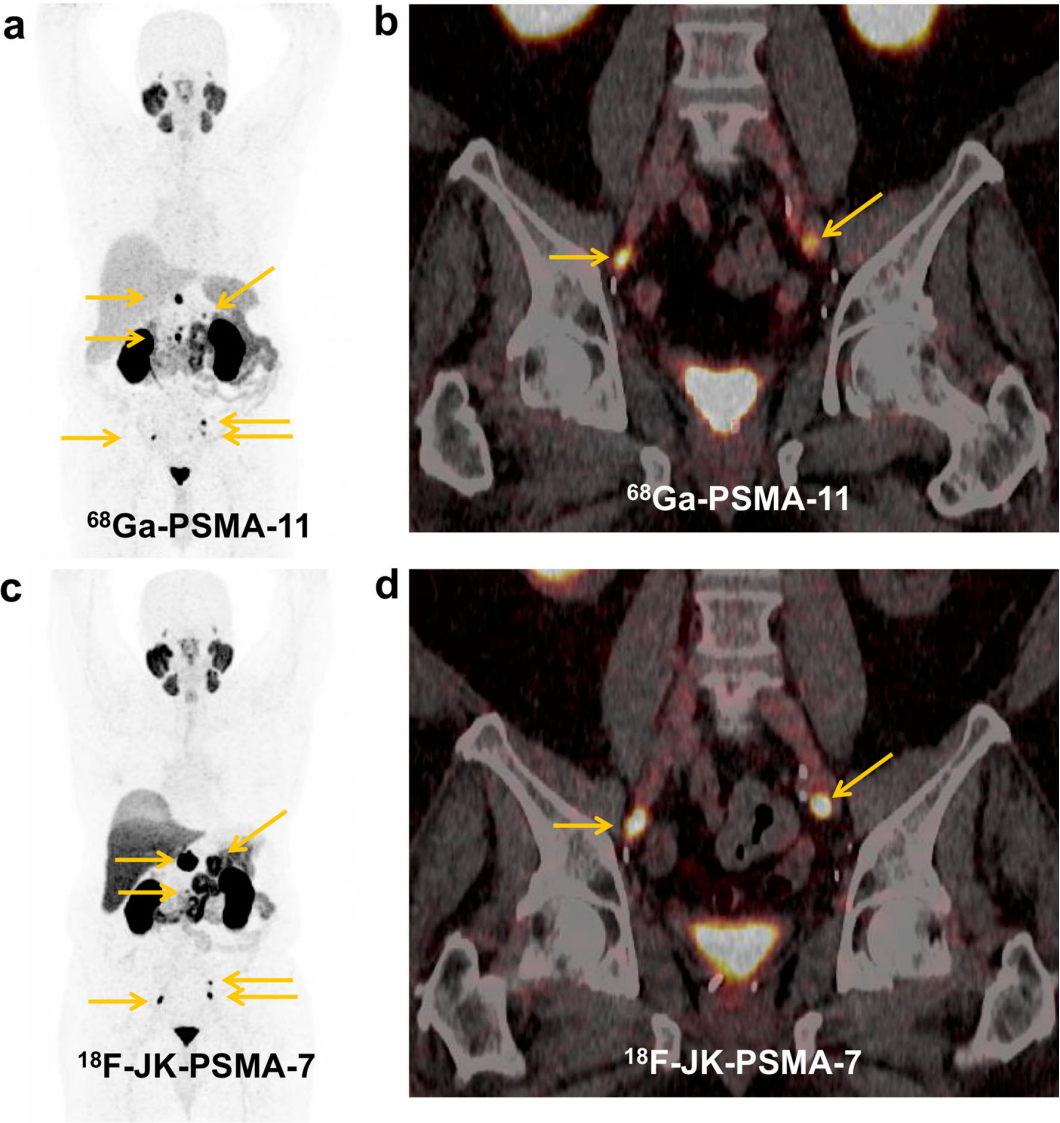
a, b Initial [^68^Ga]-PSMA-11 PET/CT (top) and c, d follow-up [^18^F]-JK-PSMA-7 PET/CT after 8 months (bottom). Concordant PSMA-positive lymph node metastases and PSMA-positive visceral metastasis in the left adrenal gland under ADT in a castrate-resistant patient with relatively low PSA levels. [^18^F]-JK-PSMA-7 PET shows progressive disease. During these 8 months, PSA levels increased from 0.2 ng/ml under ADT to 1.1 ng/ml under ADT.
